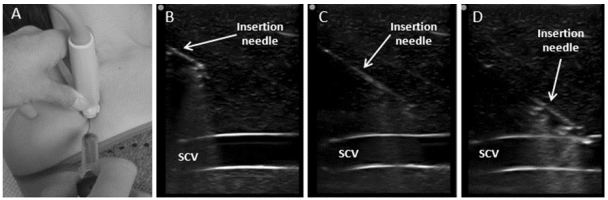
A) After identification and in-plane alignment of subclavian vein (SCV) on ultrasound, the insertion needle enters the skin at midpoint of the transducer’s small footprint and is advanced within the plane of ultrasound penetration. B), C) and D) The transducer remains in steady position enabling continuous longitudinal view of SCV, and the needle is carefully and slowly introduced with maintenance of needle visualization until the anterior wall of SCV is punctured.
